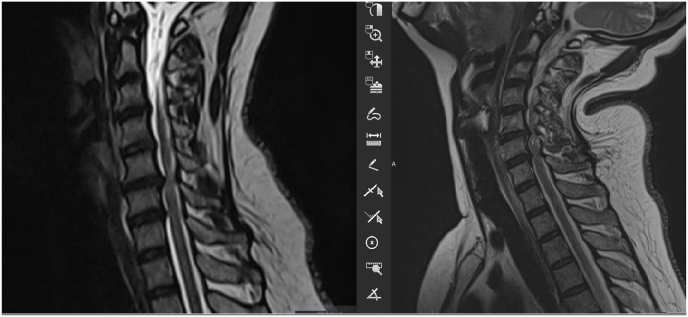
Dynamic MRI shows severe ductal stenosis in C5C6 (right) and the appearance of multiple stenosis a from C3 to C7 in extension (left).
